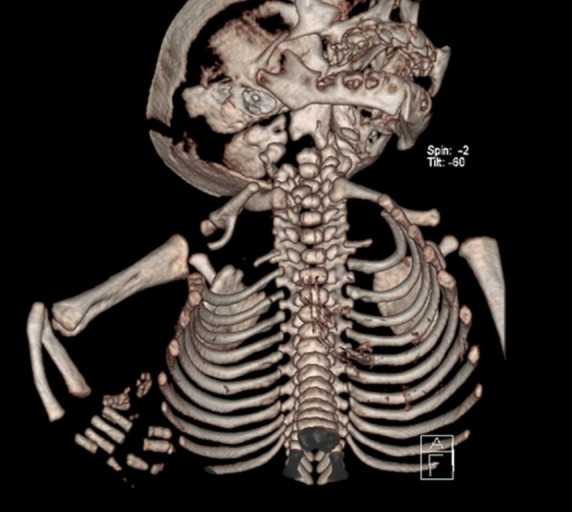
Figure 3: A)CT scan of right limb deformity.
